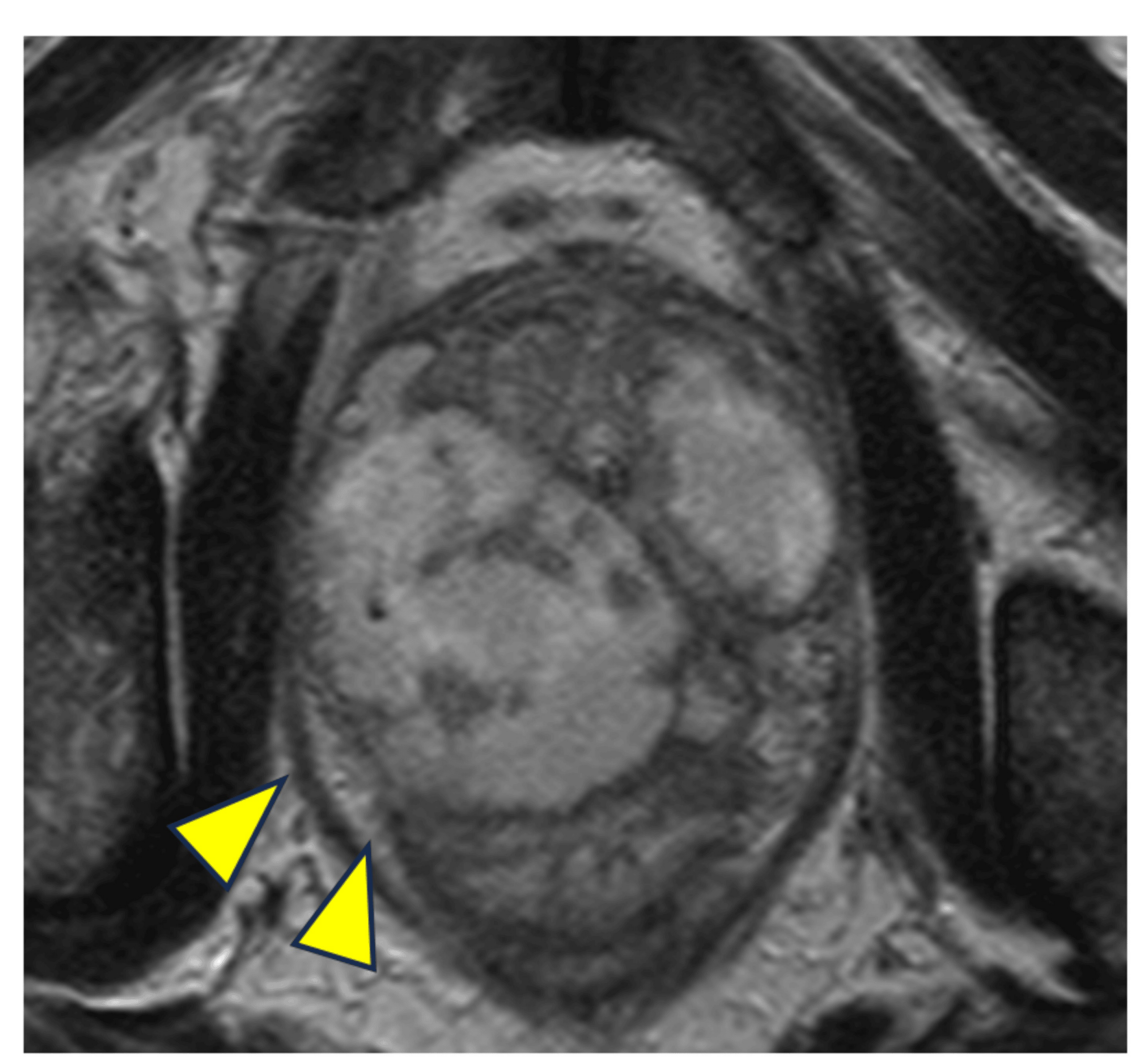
MRI of swollen prostate and seminal vesicles prior to initiation of treatment The prostate and bilateral seminal vesicles were markedly swollen, a finding that was suspicious for fluid collection in the arrowheads area.
